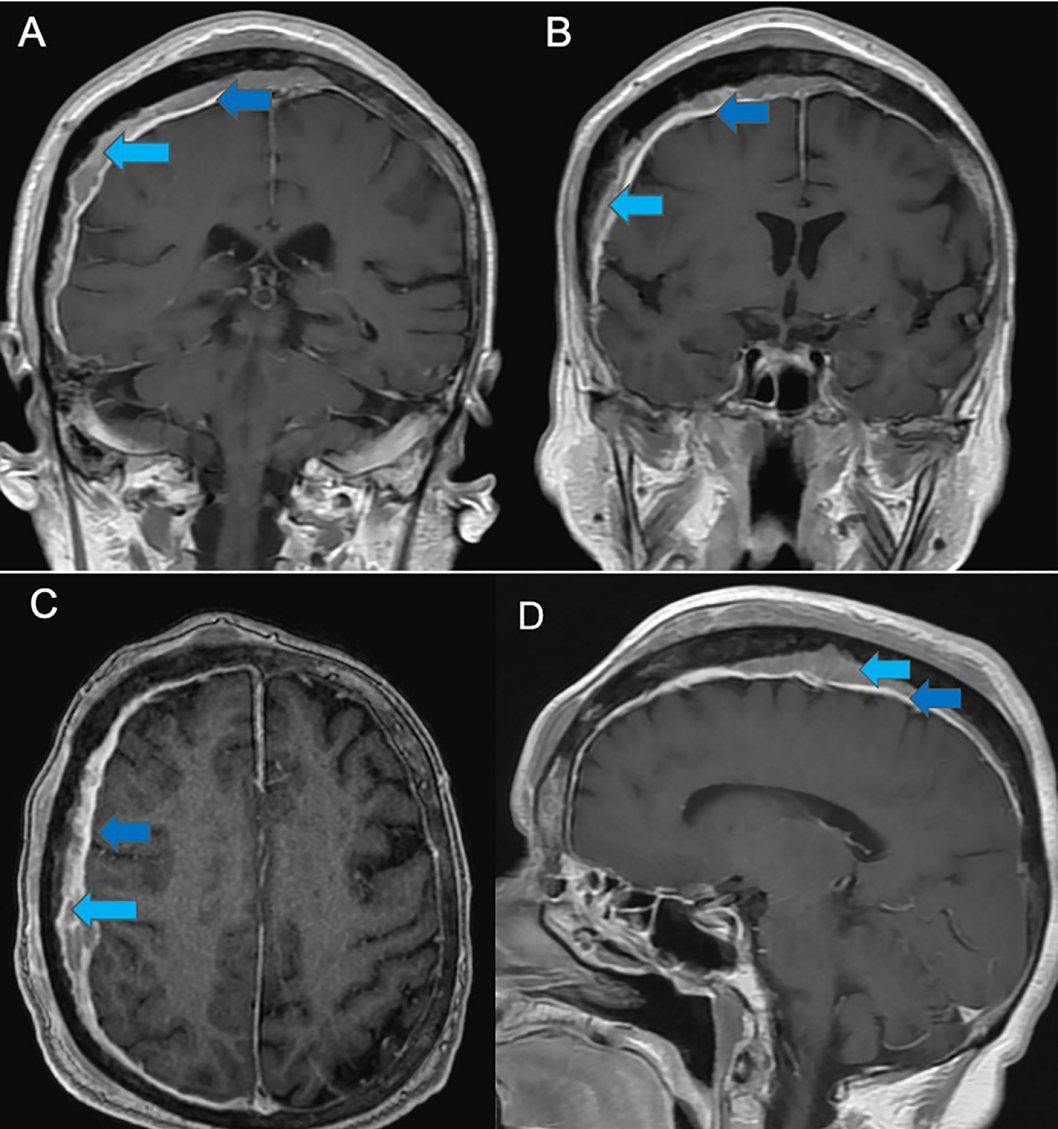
Magnetic resonance imaging of the brain, T1 sequences post contrast administration, coronal, axial, and sagittal views. (A and B) are coronal views showing near homogenous enhancement of the right subdural collection (light blue arrow) with underlying dural enhancement (dark blue arrow). (C and D) are axial and sagittal views, respectively, showing similarly.
